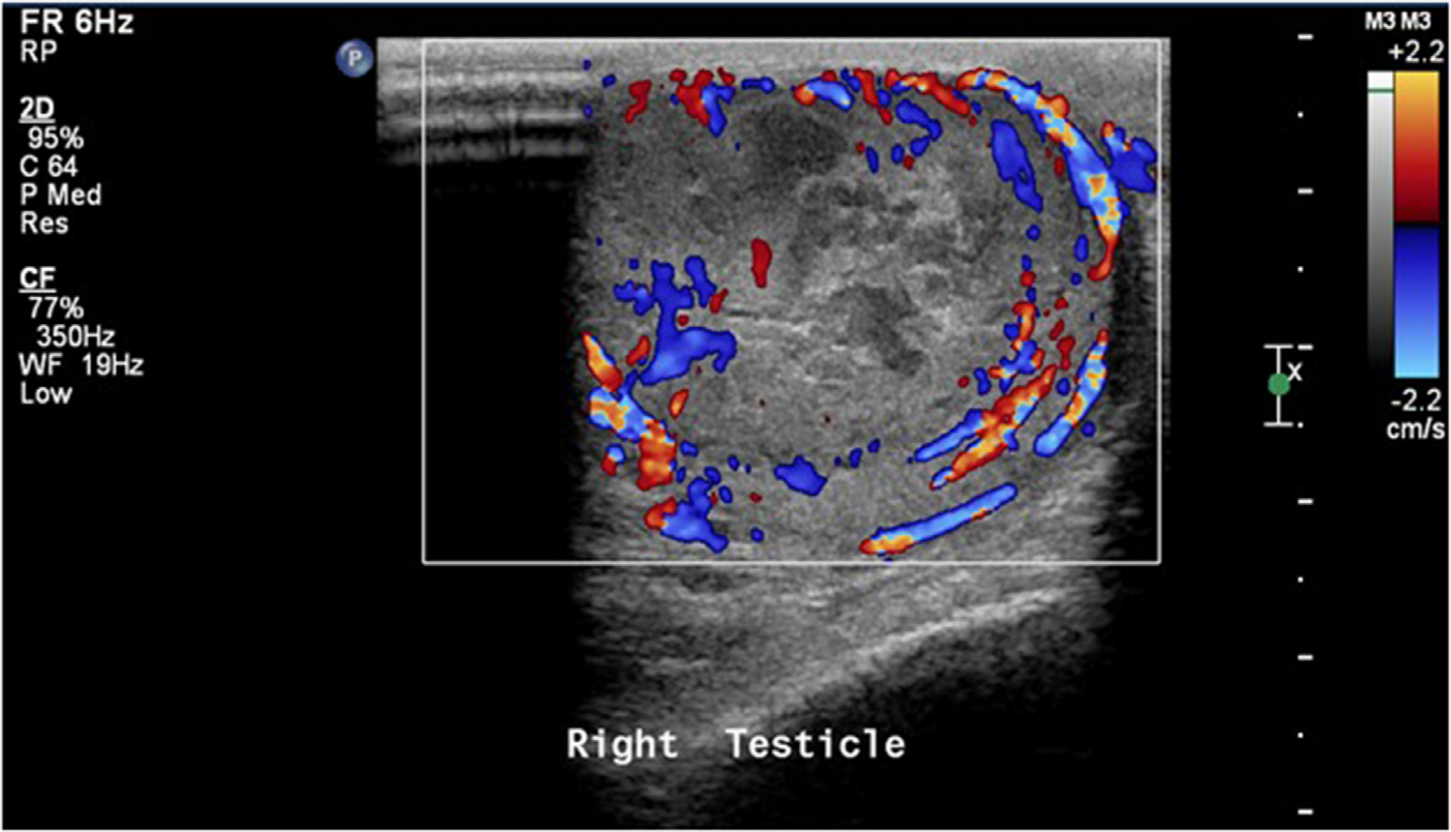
Doppler ultrasound of the same testis showing significant perilesional hyperemia denoting severe inflammation.
